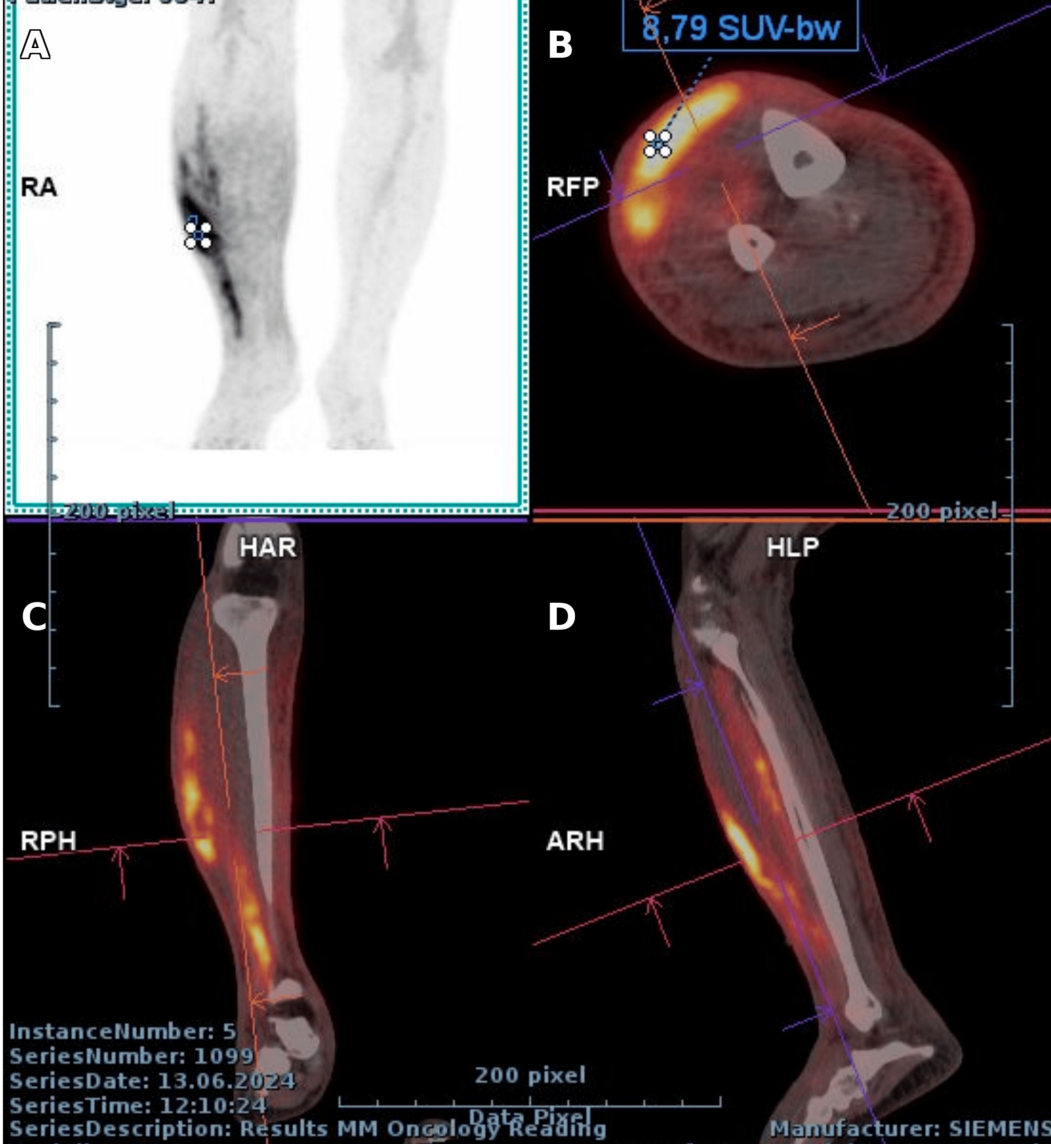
PET/CT of the right lower limb: (A) Maximum intensity projection (MIP) showing hypermetabolic focus in the right leg; (B) Ax slice with SUVmax 8.79; (C) Cor slice confirming longitudinal extension; (D) Sag slice showing distribution along the leg musculature
